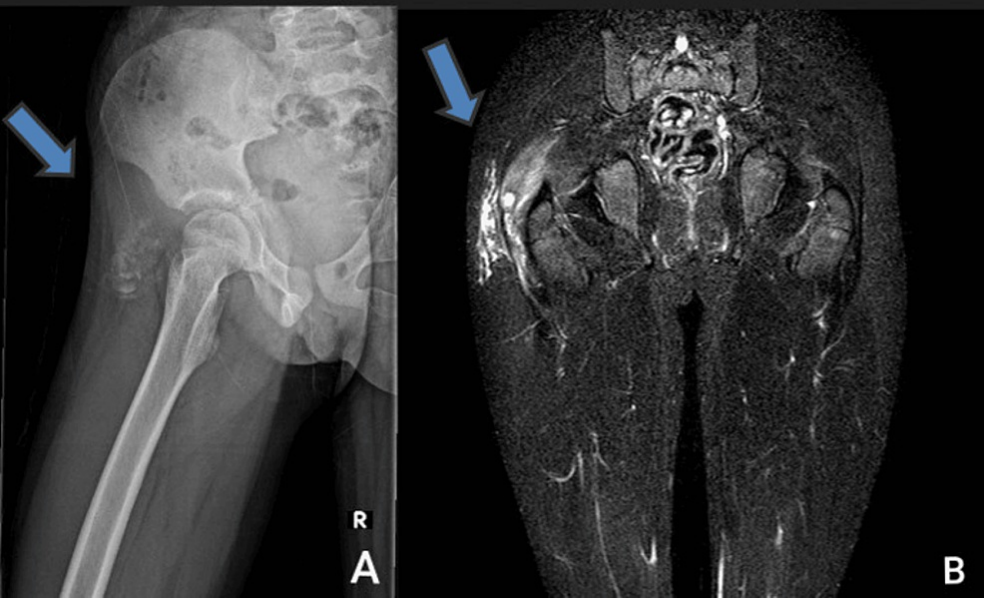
Case 2 X-ray revealed soft tissue calcifications around the right greater trochanter consistent with tumoral calcinosis (A) and MRI revealed a calcified right hip mass (B)
